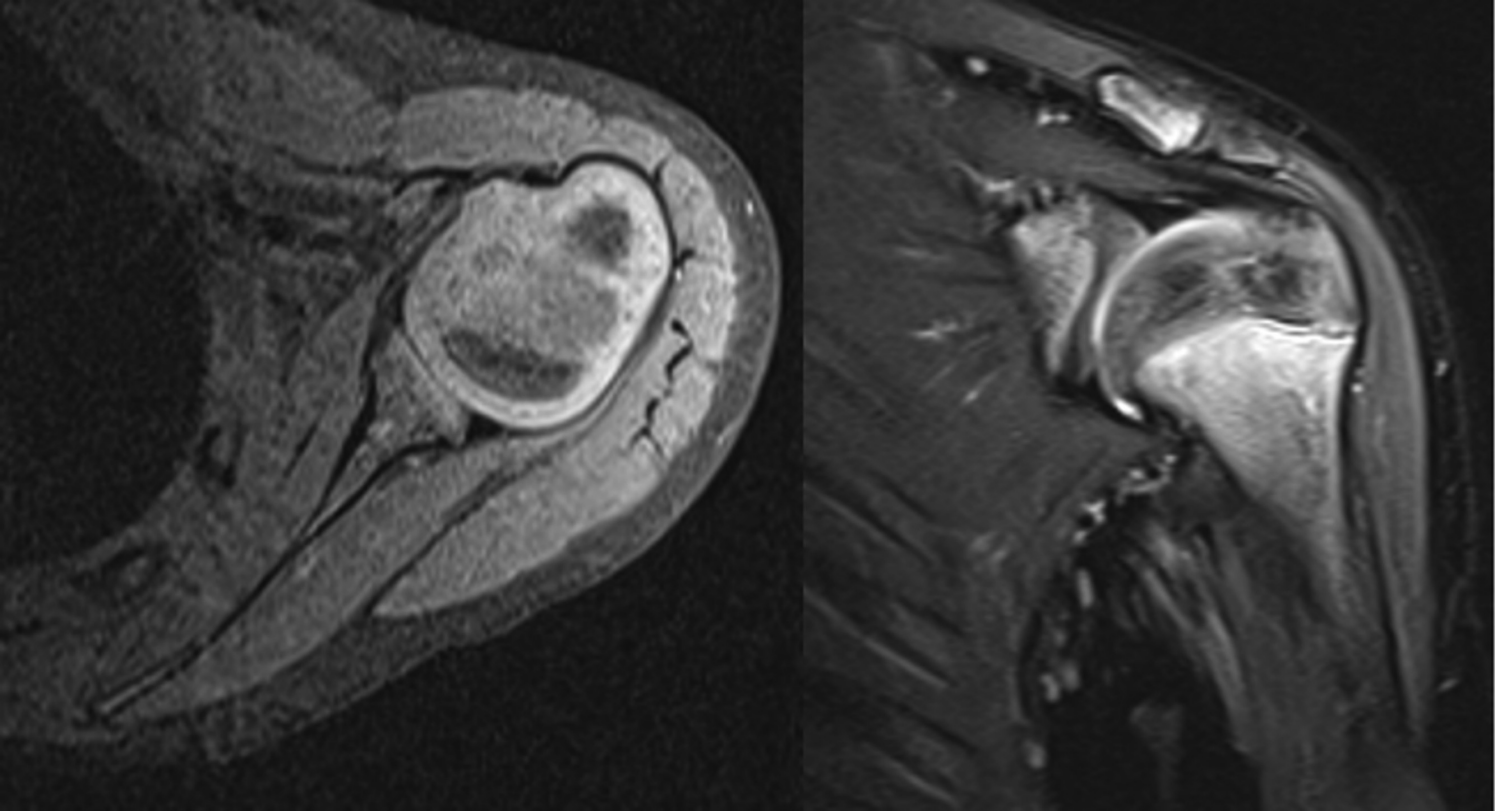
Representative magnetic resonance imaging (MRI) of glenohumeral joint in patient with global brachial plexus injury.
